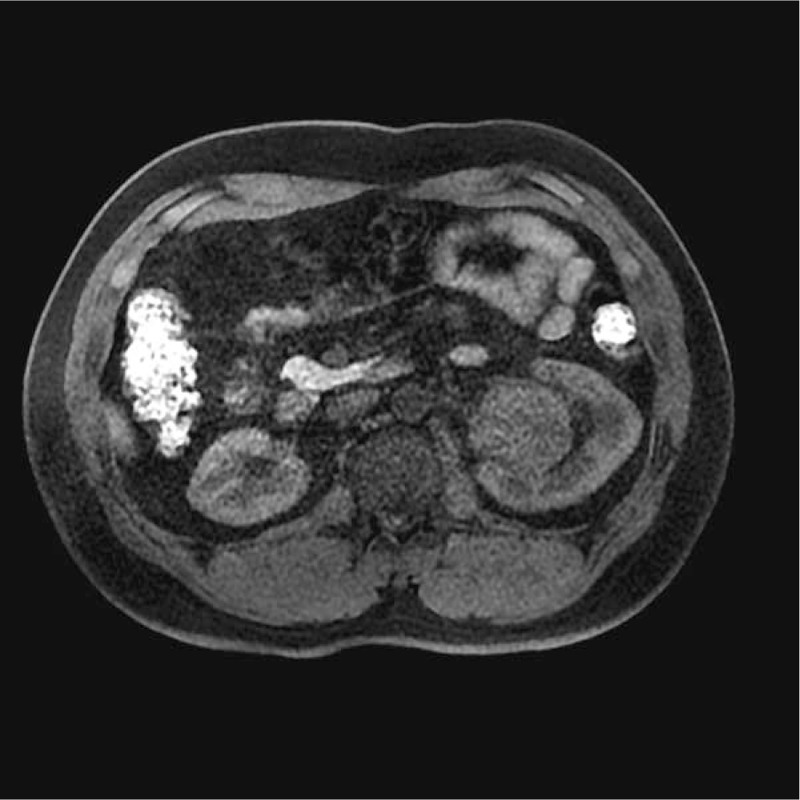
On precontrast T1-weighted dual-echo image, the lesion showed isointensity compared with the renal cortex.
